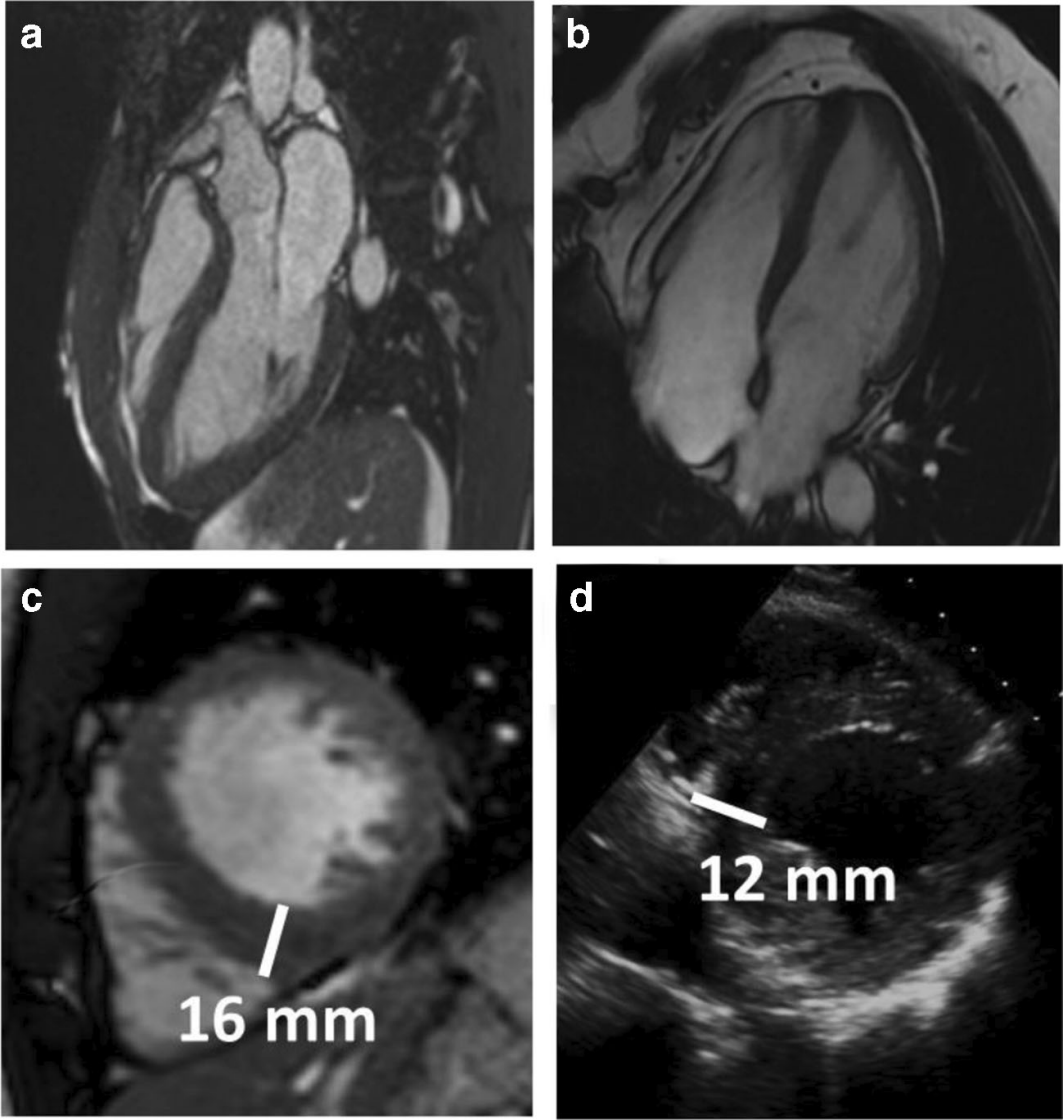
Focal hypertrophy potentially missed by echocardiography. Two athletes presenting with focal hypertrophy limited to the apex (a, b). c Short axis view by CMR showing focal hypertrophy limited to the inferior septum with a maximum wall thickness (16 mm) measured at the RV insertion point in an athlete. d The corresponding short-axis view by echocardiography underestimated the hypertrophy (12 mm)
